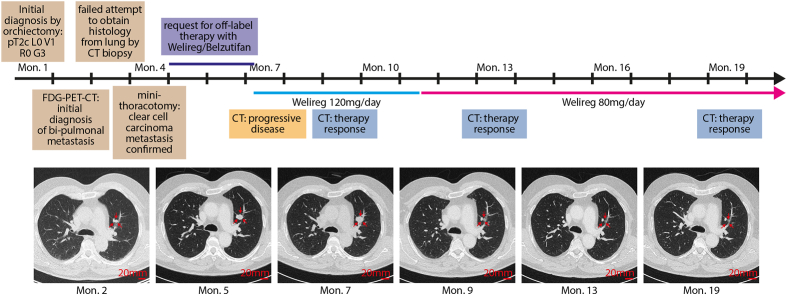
Timeline and exemplary CT-scan-slices for the treatment response.
